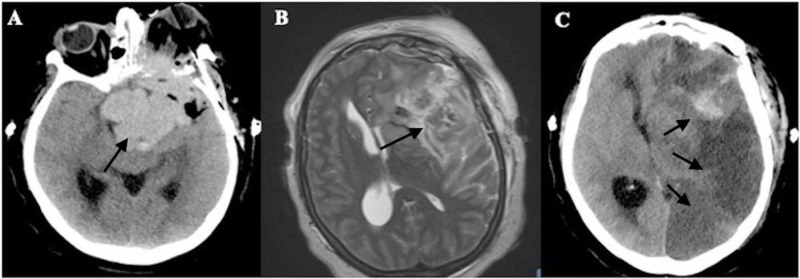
Postoperative imaging. (A) Non-contrast CT scan on postoperative day 0 showing decreased tumor size. (B) T2-weighted MRI scan on postoperative day 2. Axial view showing new area of infarction in the left middle cerebral artery (MCA) distribution. (C) Non-contrast CT scan on postoperative day 6 showing worsening left MCA infarction and left to right midline shift.
